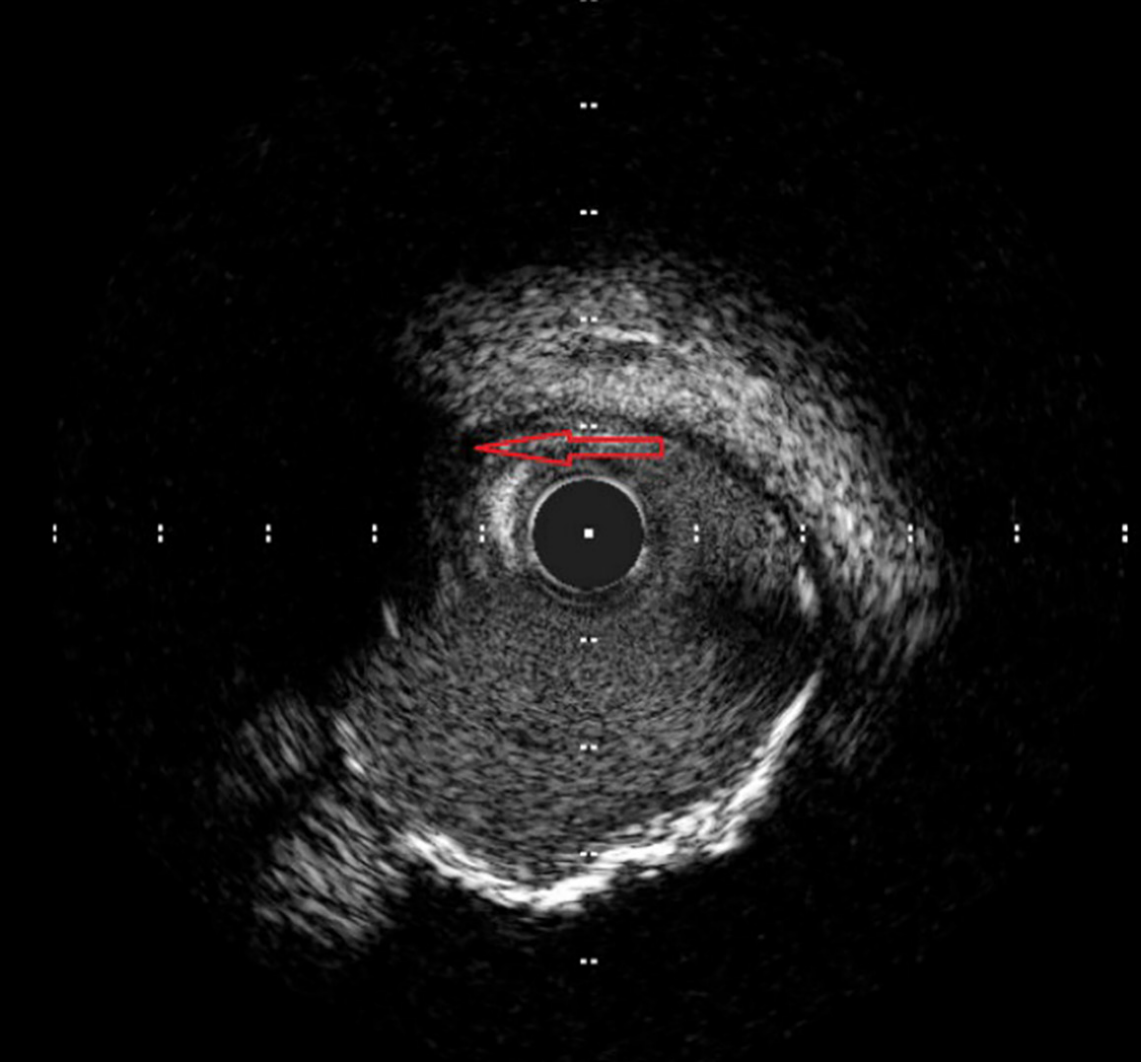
Intravascular ultrasound showing plaque with rupture (red arrow).
